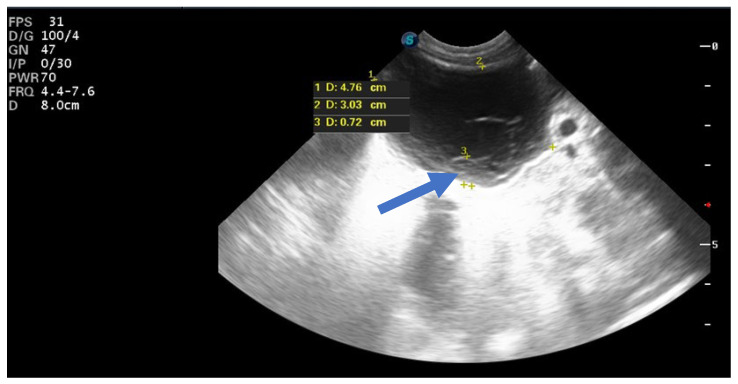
Ultrasound image of a neonatal simple cyst with a daughter cyst (blue arrow), which regressed spontaneously after 2 months.
